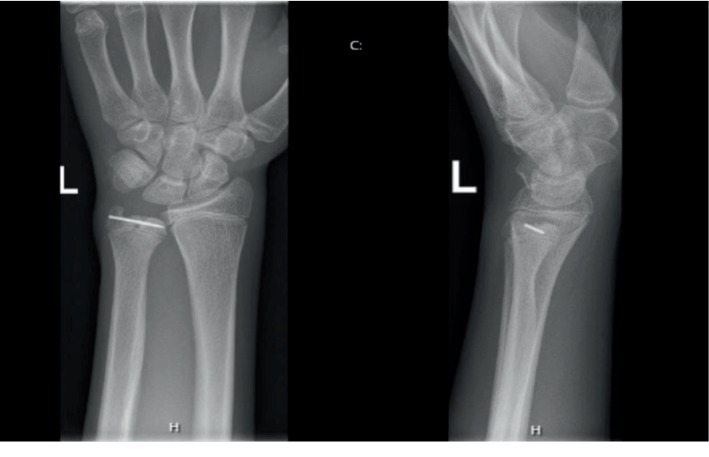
Anteroposterior and lateral X-ray 6 months after surgery. Two pins have been removed. No physeal closure visible.
